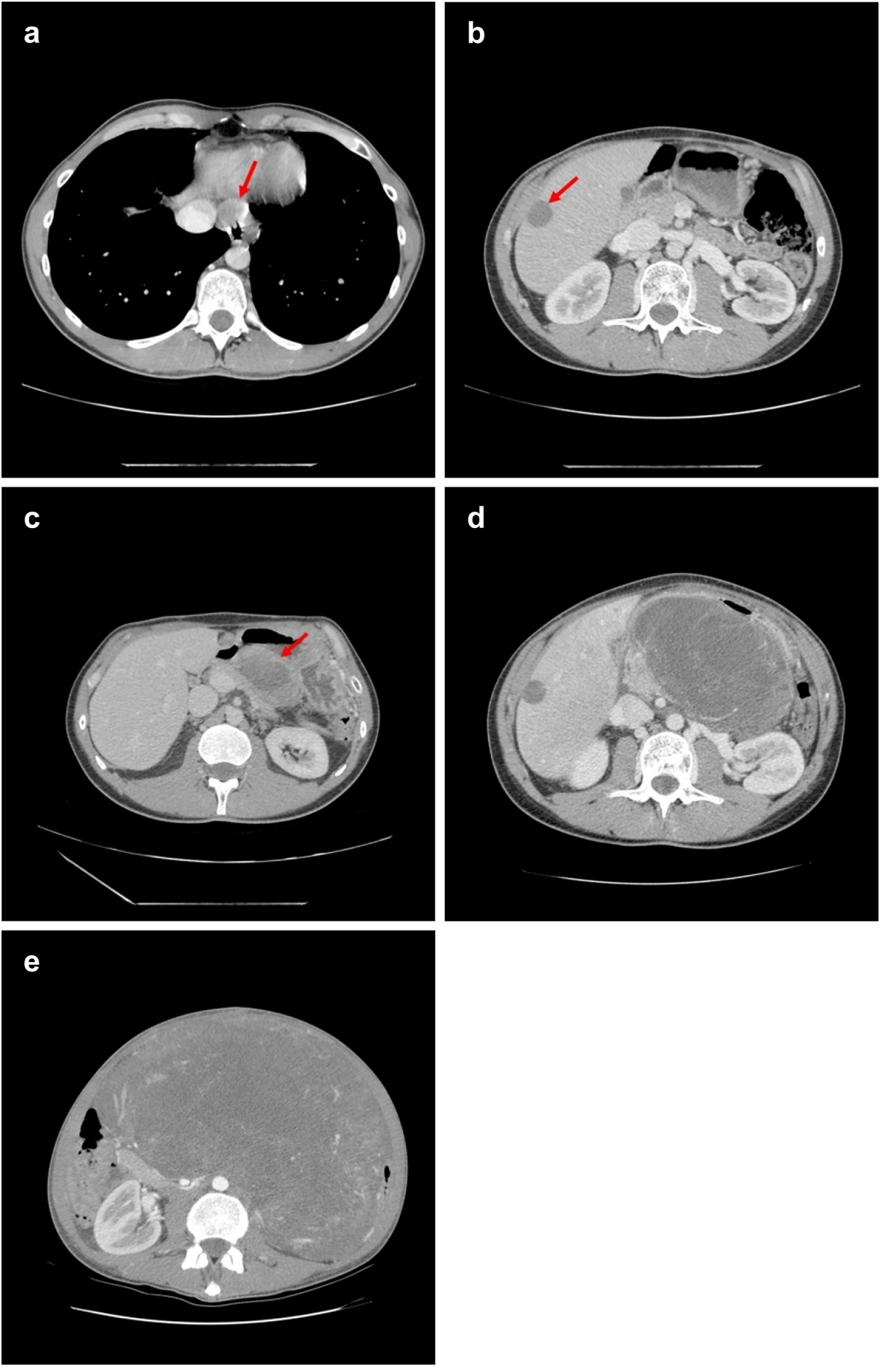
CT imaging of the patient under treatment. a, b Follow-up CT scan from September 2017 shows a partial response to imatinib, with reduced size of periesophageal and hepatic lesions (red arrows). c By February 2018, CT imaging reveals disease progression, with new intra-abdominal lesions, including an 8 cm perigastric mass with central necrosis (red arrow). d Following imatinib dose escalation, further progression is observed, including enlargement of the perigastric mass. e Imaging obtained at the late-stage of disease showing a large tumor mass occupying most of the abdominal cavity.
